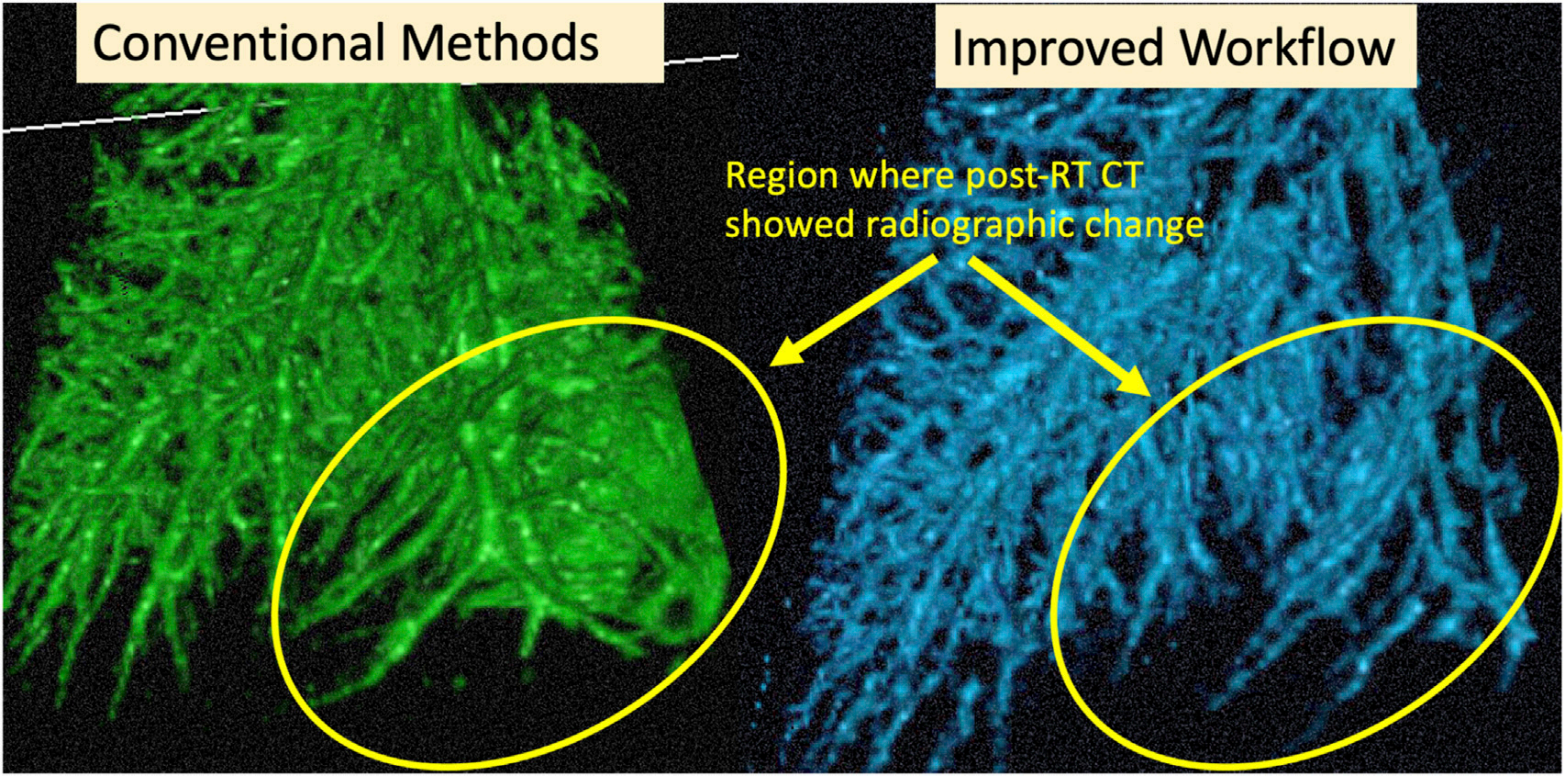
Example cropped 3D Rendering of an example subject. Circled regions show where the CT showed radiographic change post-RT. We see the result of the over-segmentation in the conventional method and the improvement on this using the proposed method.
